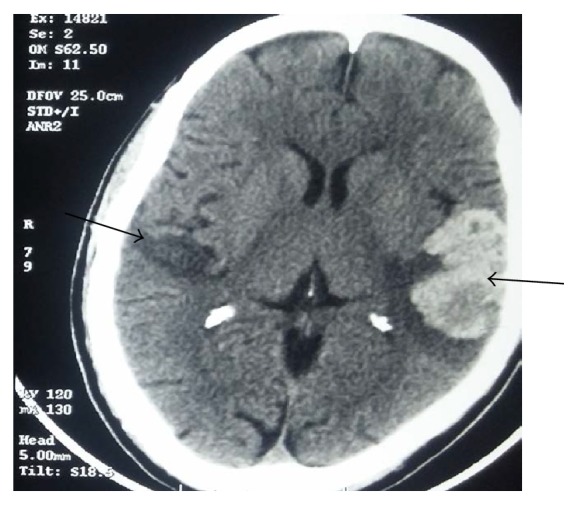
CT head showing infarcts in bilateral temporal lobes with haemorrhagic transformation on left.
